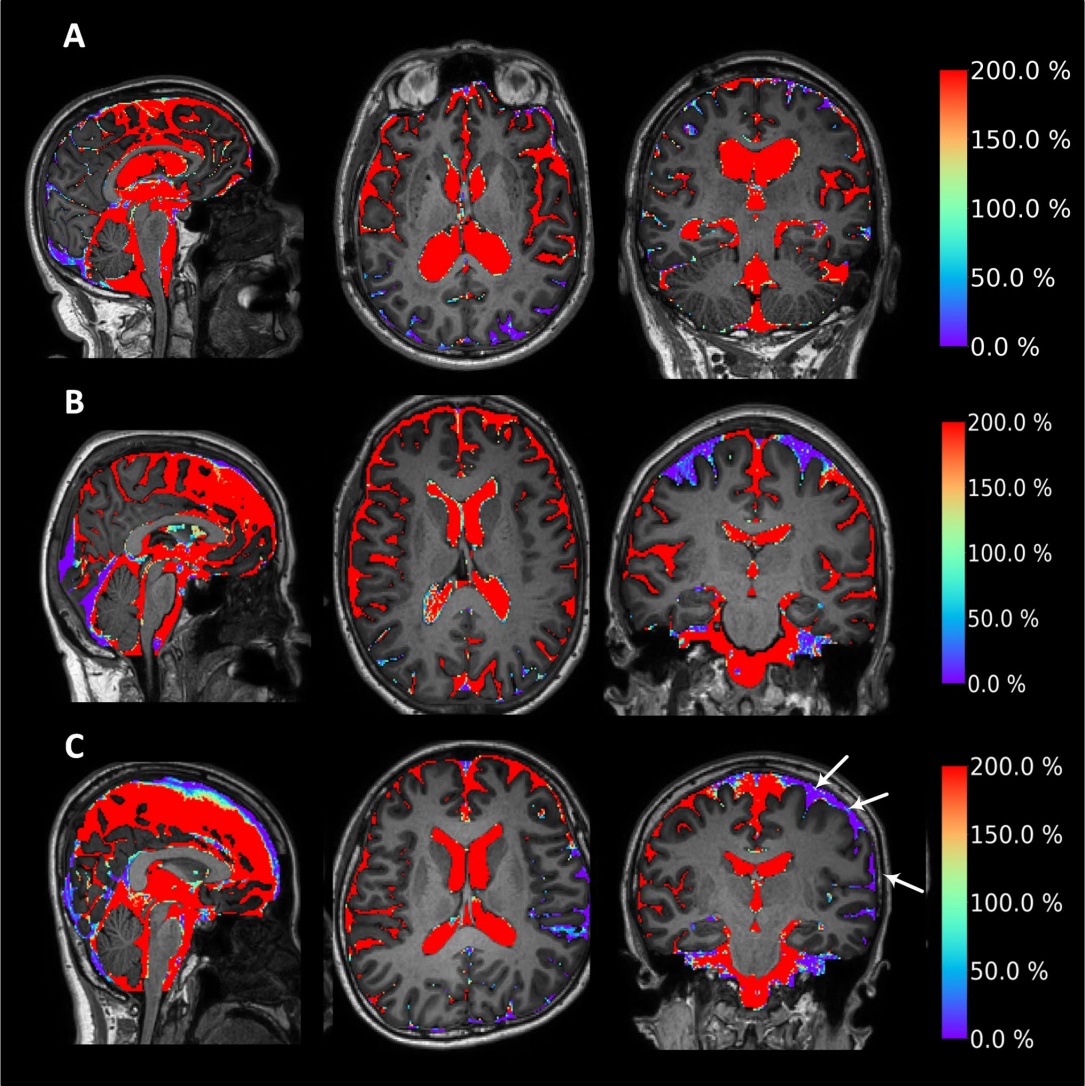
Impact of cerebral bleed on tracer enrichment in subarachnoid spaces and ventricles 24 h after intrathecal tracer injection. Tracer enrichment in the subarachnoid spaces was symmetrical between (A) the affected and unaffected hemispheres of the patient with acute subdural hematoma (aSDH) 2.8 months earlier and between (B) the left and right hemispheres of a reference (REF) subject. In contrast, C tracer enrichment was reduced on the affected side (white arrows) in a subject with asymmetrical subarachnoid hemorrhage (SAH) 6.3 months earlier (i.e., reduced tracer enrichment on affected side). All three cases exhibited strong ventricular tracer enrichment at 24 h. The degree of tracer enrichment 24 h after intrathecal injection is displayed in sagittal (left column), axial (middle column), and coronal (right column) MR images. The percentage increases in tracer enrichment compared to baseline are indicated on the color bar to the right
